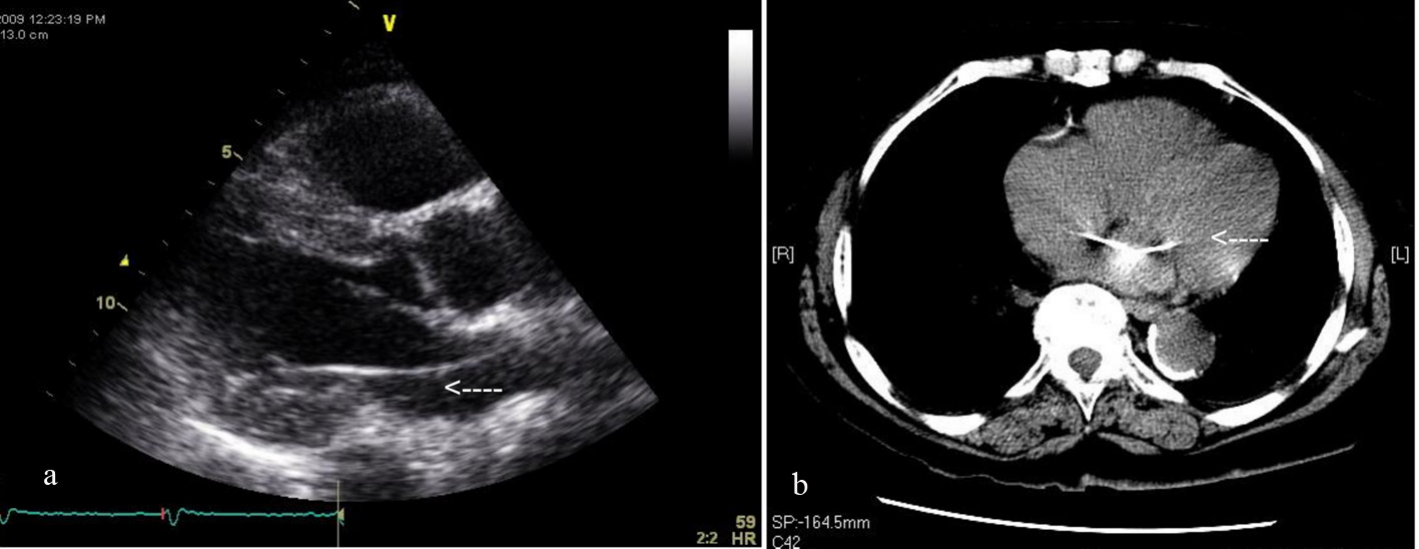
a: echocardiogram showing the presence of the ventricular pacing lead in the left ventricle; b: CT scan of the chest demonstrating the ventricular lead crossing the inter-atrial septum.
